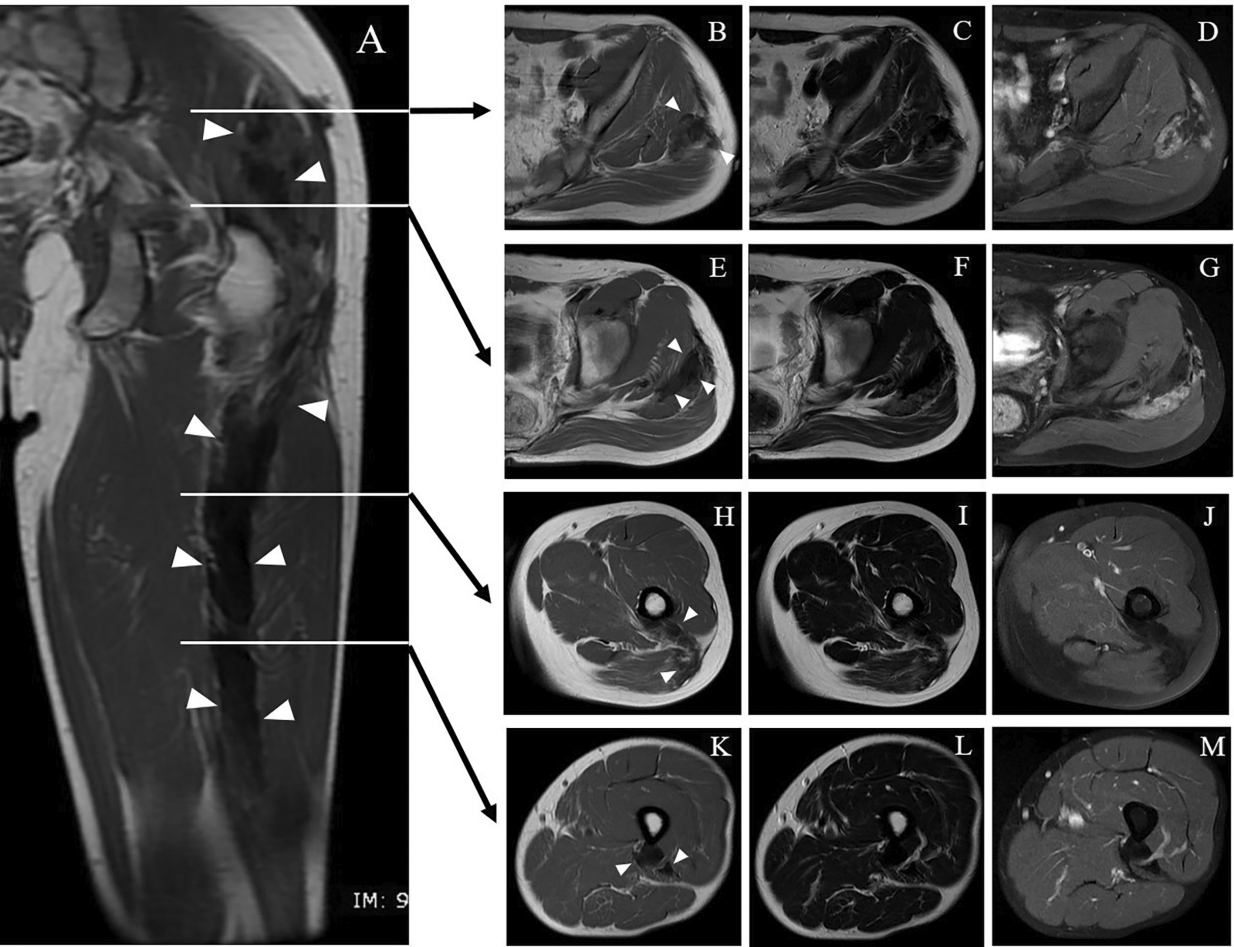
Thirty‐one‐year‐old male patient. Magnetic resonance images of the left hemipelvis show homogeneously low‐intense lesions on T1‐, T2‐, and Gd‐contrast enhancement fat suppression T1‐weighted images of the left gluteus maximus and gluteus medius muscles, extending to the vastus lateralis and biceps femoris. The maximum diameter of the lesion is 35 mm. The tumor is indicated by arrowheads. (A) T1WI coronal sequence. (B, E, H, K) T1WI axial. The tumor is shown using arrowheads. (C, F, I, L) T2WI axial sequence. (D, G, J, M) Gd‐contrast enhancement fat suppression T1WI.
